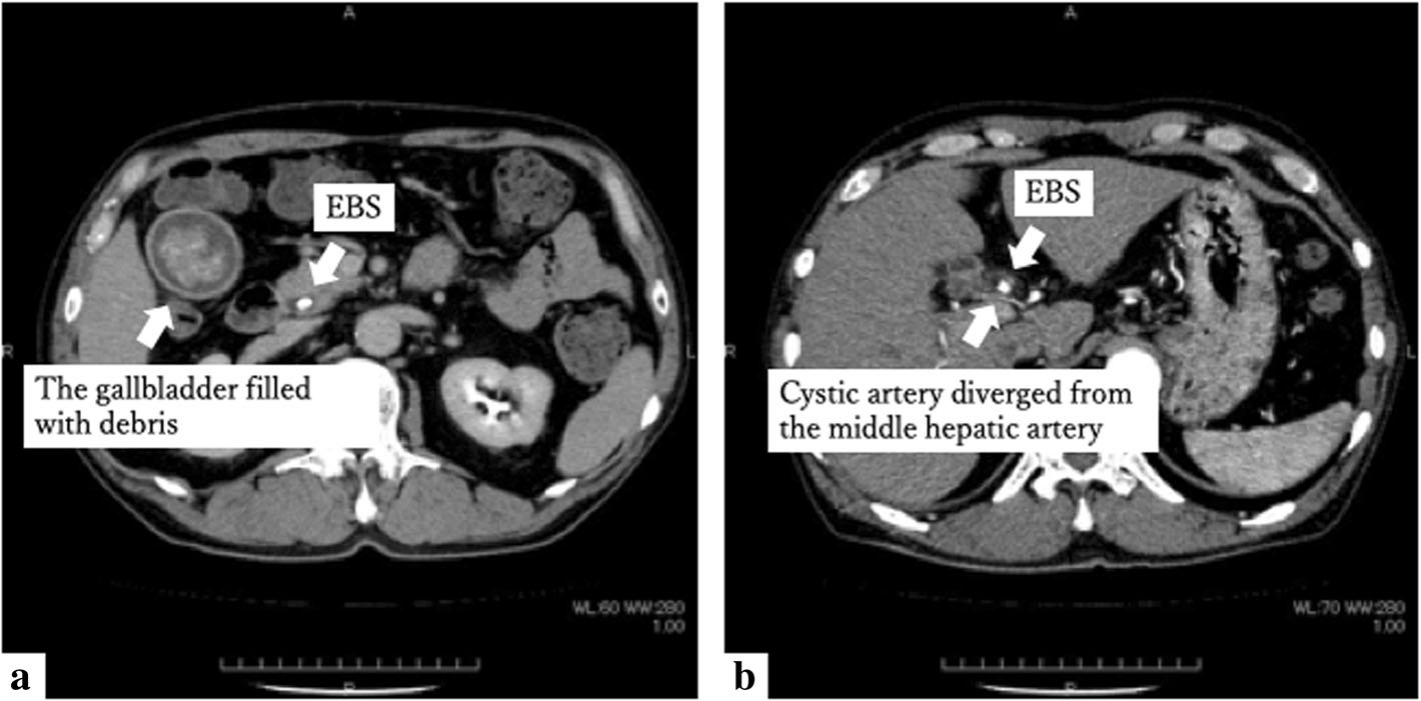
Contrast CT before LC. a The gallbladder was filled with debris. b Cystic artery diverged from the middle hepatic artery
